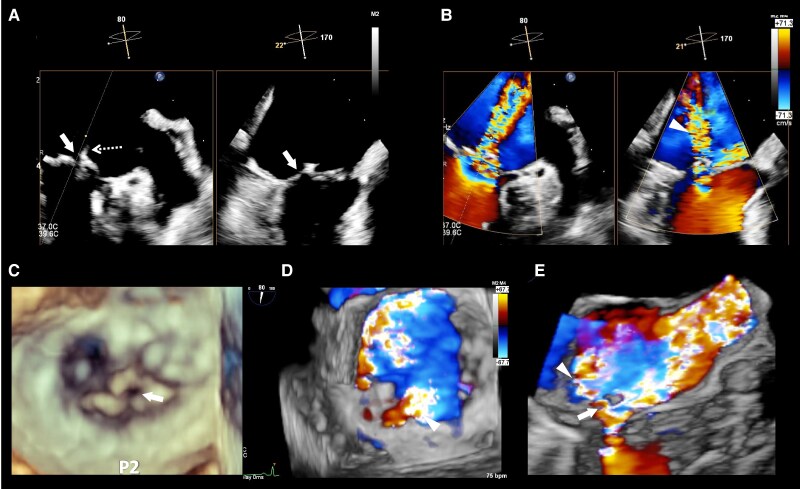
Pre-operative transoesophageal echocardiography (TOE). (*A*) Simultaneous visualization of two orthogonal planes showing the tissue discontinuity of posterior leaflet (arrow) and a string-like echo (braille arrow) on the left atrial side suggested a perforation of the posterior leaflet. In the same plane as (*A*), a jet directed posteriorly towards the left atrial roof (arrowhead) is observed at the perforation site on colour Doppler imaging (*B*). The P2 perforation determined in 3D TOE image (*C*). On 3D colour Doppler imaging, MR caused by P2 prolapse blowing anteriorly and a jet blowing posteriorly through the perforation were observed separately (*D*, *E*). Graphical elements; arrow = perforation of posterior leaflet, braille arrow = string-like echo on mitral valve nearby the perforation, arrowhead = MR jet towards the backward due to the perforation. MR, mitral regurgitation; P2, the middle segment of the posterior leaflet.
